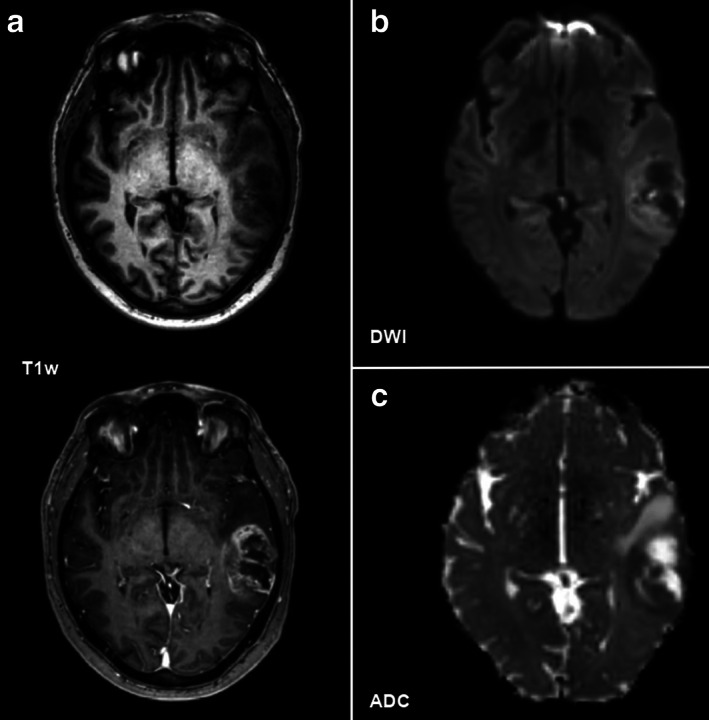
Diffusion restriction in a 45‐year‐old female patient with a left temporal high‐grade glioma (HGG) that showed contrast enhancement on T1‐weighted imaging (a; axial non‐contrast and contrast‐enhanced images). On diffusion‐weighted imaging (DWI, *b* = 1000 s/mm^2^), the borders of the lesion that primarily overlap with the contrast‐enhancing tumor parts show high signal intensity (b) that spatially corresponded to areas with a signal drop on apparent diffusion coefficient (ADC) maps (c), indicating restricted diffusion most probably due to focal high cellularity of the glioma.
